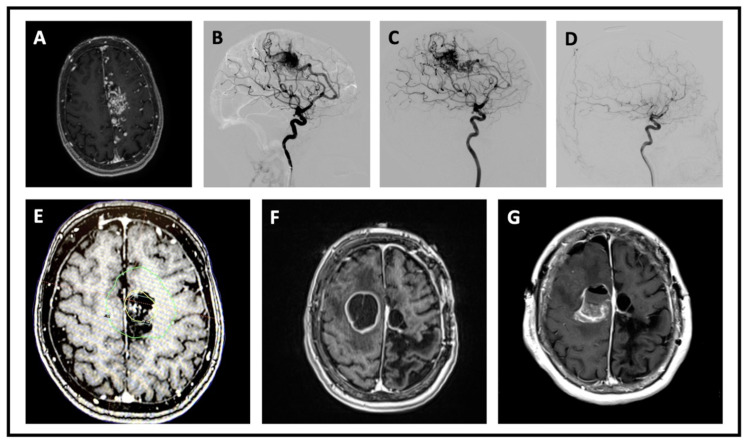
A: Axial T1 MRI with contrast demonstrating left-sided parasagittal AVM. B: Lateral digital subtraction angiography demonstrating AVM arising from the distal branches of the anterior cerebral artery. C: AVM status post-embolization. D: AVM status post-resection. E: Axial CT of the head with contrast demonstrating SRS treatment plan for left-sided parasagittal AVM; the yellow outline shows the target within the dose prescribed (23 Gy at the 50% isodose), the green line represents the 12 Gy line. F: Axial T1-weighted MRI with contrast demonstrating right-sided parasagittal glioma adjacent to the previously radiated and resected AVM on the contralateral side. G: Axial T1-weighted MRI with contrast demonstrating right-sided parasagittal glioma status post-resection. AVM: arteriovenous malformation; CT: computed tomography; MRI: magnetic resonance imaging
